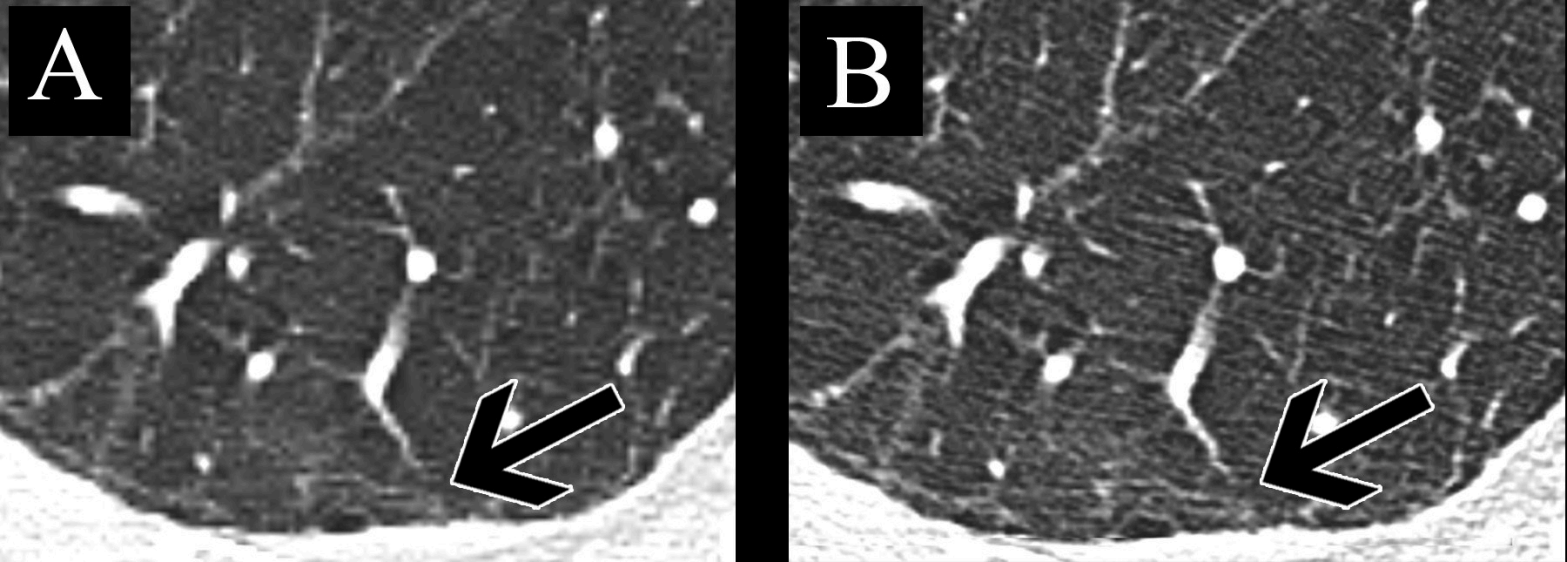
Image examples of a 67-year-old man imaged for interstitial pneumopathy. Axial CT slice reconstructed with a 512-matrix (A) and 1024-matrix (B). Fibrotic changes (black arrow) appear to be sharper in B compared to A. Objective and subjective image noise are inferior in B.
